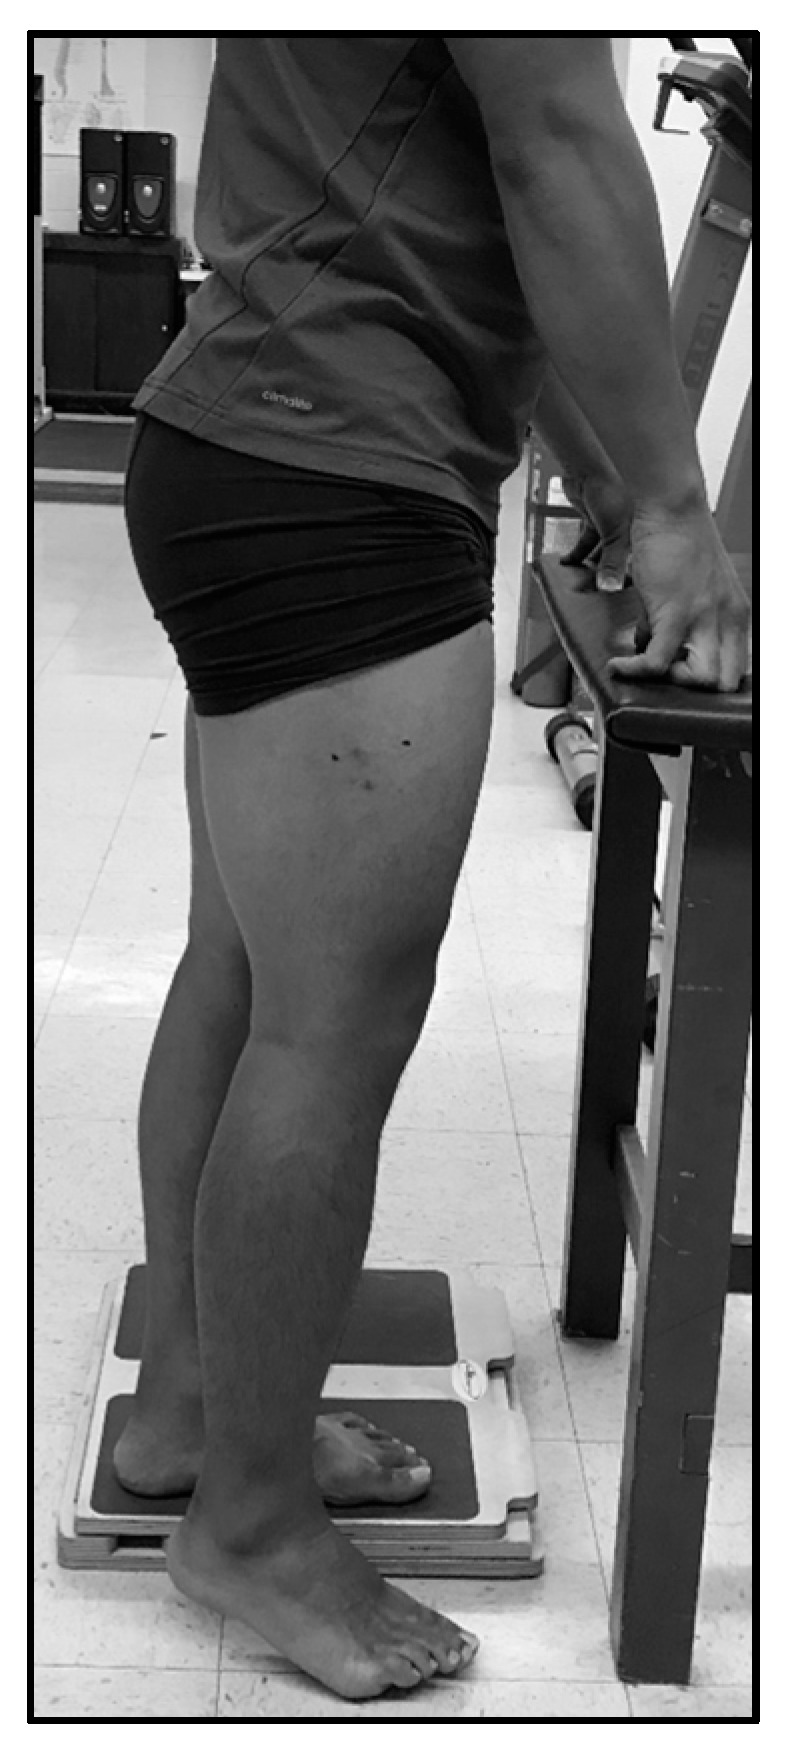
Standing ultrasonography collection position.
